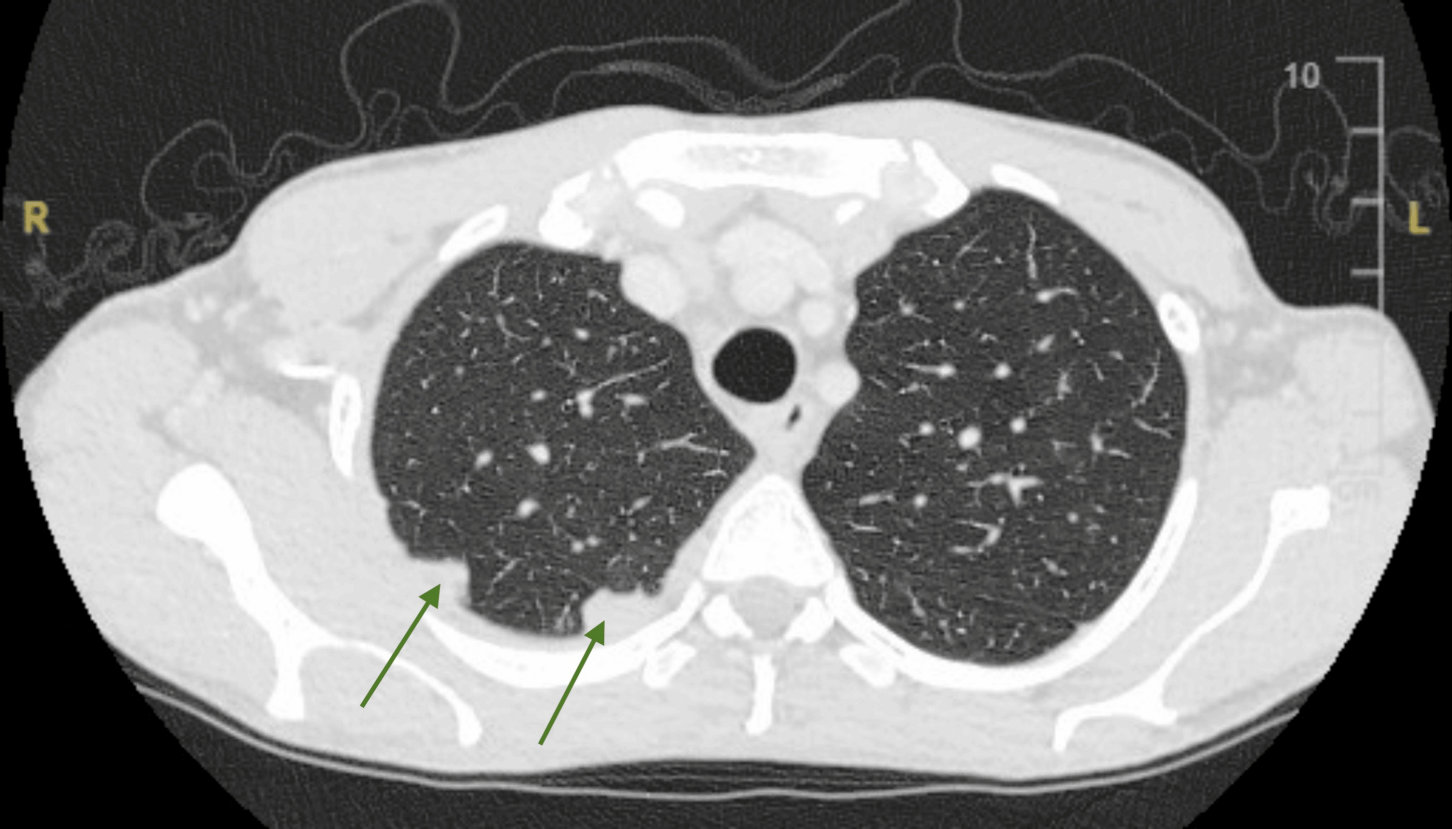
CT Thorax (lung window), axial view, showing two pleura-attached nodule opacities in the right apex posteriorly (arrows)
